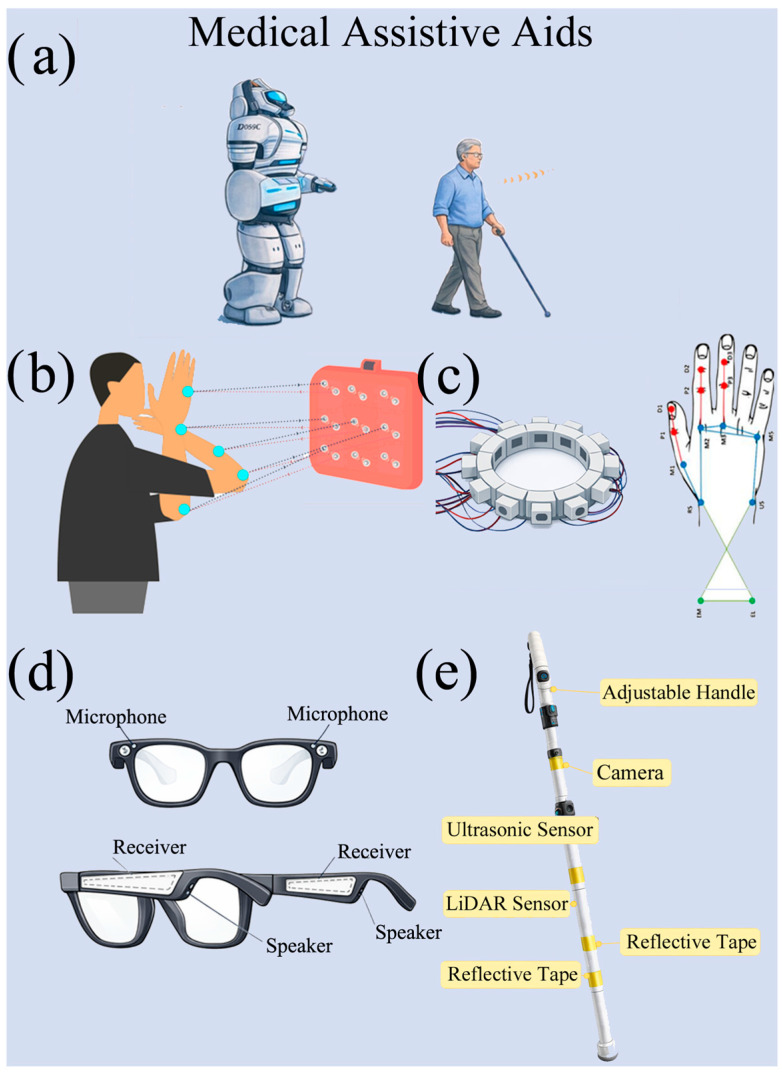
(a) Ultrasonic assistive aids and medical robotics, including obstacle detection and navigation in unstructured environments using biosonar-inspired time-of-flight sensing, applied to devices such as smart canes, wearable sensors, and mobile assistive aids. (b) Ultrasound-based gesture recognition contactless system (adapted from [55]). (c) Wearable ultrasound (bracelet) for wrist and hand kinematic tracking (adapted from [56]). (d) Smart glasses with integrated left/right microphones, receivers, and speakers adapted from [57,58]. (e) Smart cane with integrated sensing modules and user-feedback elements (adapted from [59,60]).
